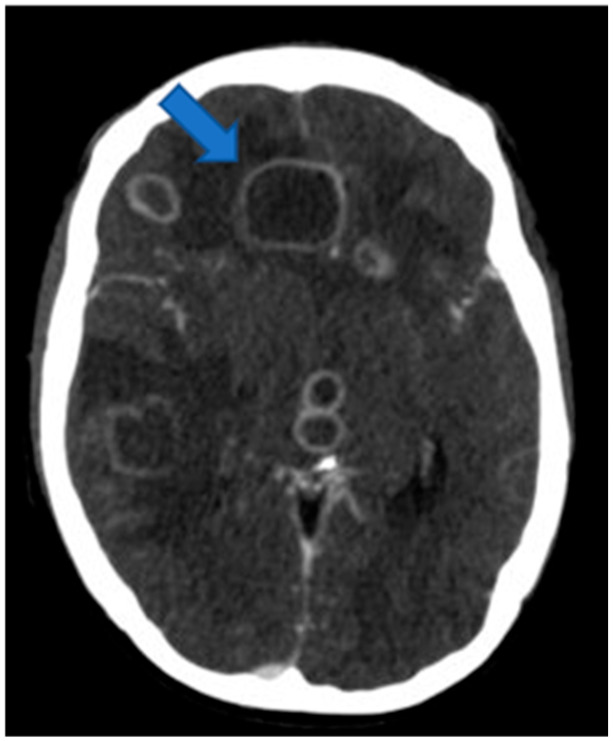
Baseline Axial CT scan: multiple hypodense nodular lesions with marked peripheral contrast enhancement, varying in size; the largest one located in the right paramedian frontal region (Figure 1, arrow).
